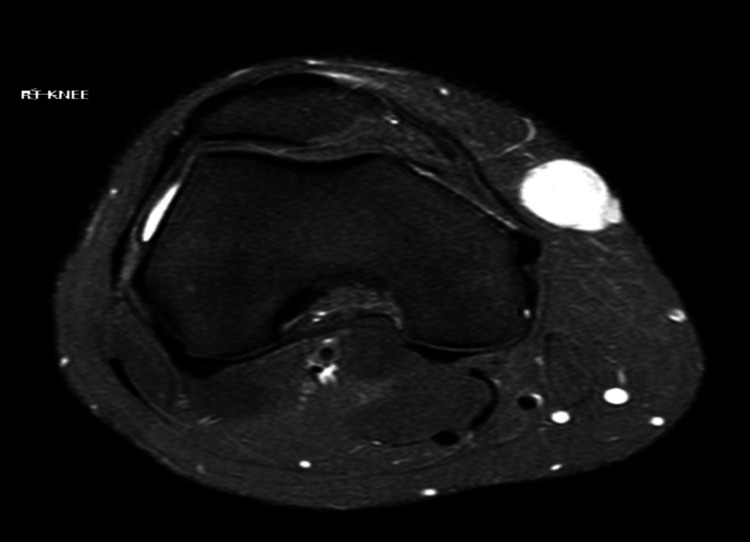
Superficial CD34-positive fibroblastic tumor: MRI of the knee. The image shows a well-defined 2.4 × 1.8 cm subcutaneous mass with no definite communication with the underlying ligament or meniscus.
